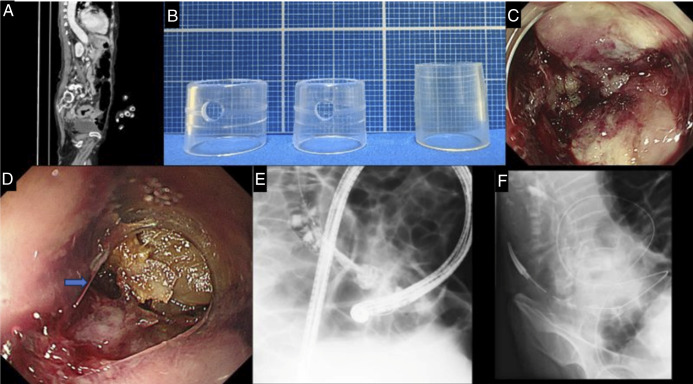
Clinical course of malignant large bowel obstruction because of sigmoid colon cancer and management using cap-assisted endoscopy and transanal decompression tube. (A) Pretreatment axial abdominal computed tomography image showing marked colonic dilatation proximal to the obstructive lesion in the sigmoid colon, suggestive of malignant large bowel obstruction. (B) Endoscopic caps used during the procedure. Left: short cap (4-mm protrusion; D-201-12704, Olympus); center: short cap (4-mm protrusion; d-201-13404, Olympus); right: long cap (7-mm protrusion; Space Adjuster, TOP, Tokyo, Japan). (C) Endoscopic view of the anal side of the tumor using a short cap. The lumen is not identifiable. (D) Endoscopic view of the anal side of the tumor using a long cap. The lumen is clearly visualized, facilitating scope alignment. Arrow indicates the lumen. (E) Fluoroscopic image after successful guidewire placement across the stricture. (F) Fluoroscopic image following placement of a transanal decompression tube, showing decompression of the dilated colon.
